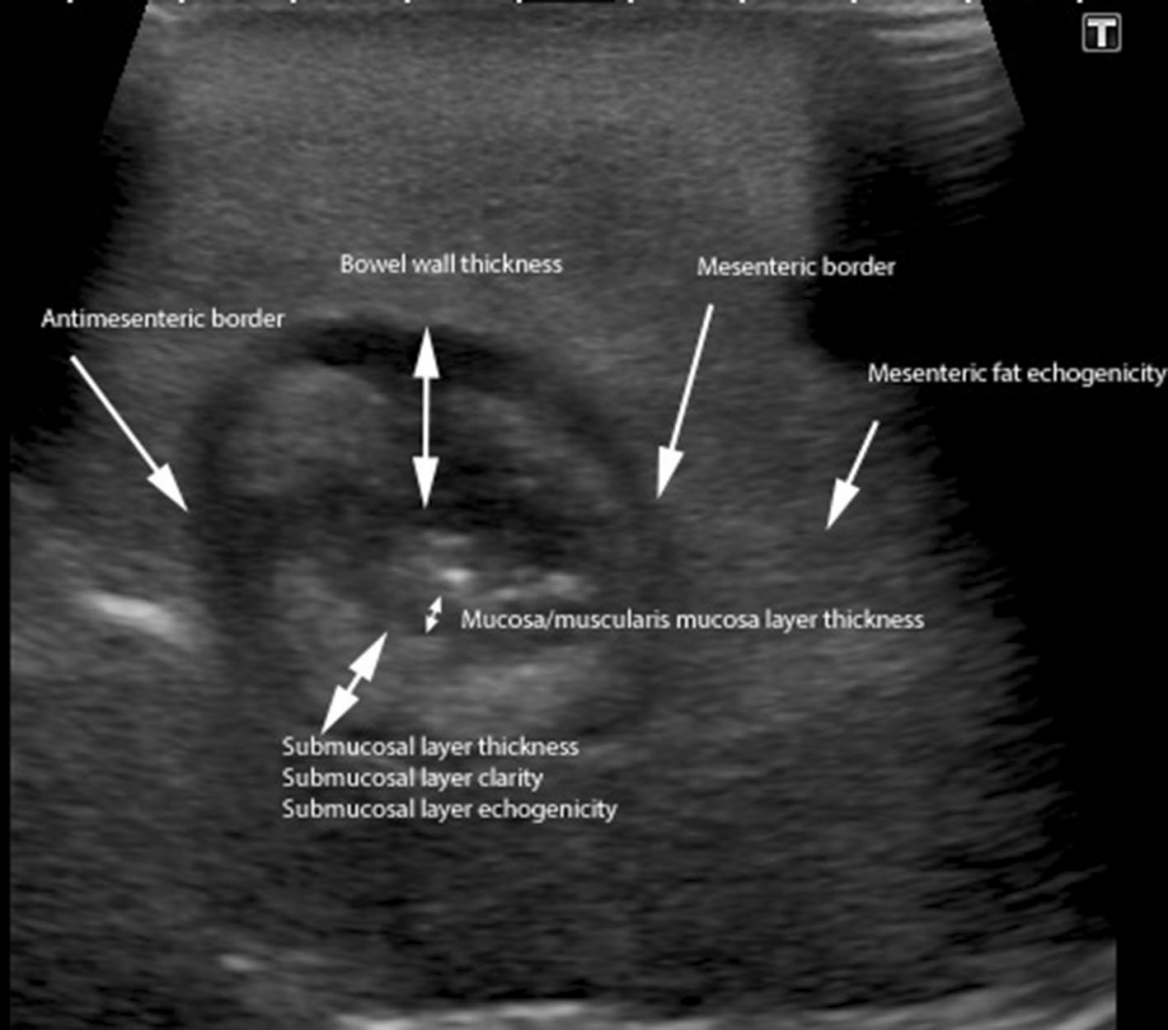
B-Mode US demonstrating multiple variables evaluated. Additionally, the presence of ulceration, increased Doppler vascular pattern and stenosis causing functional obstruction were recorded
